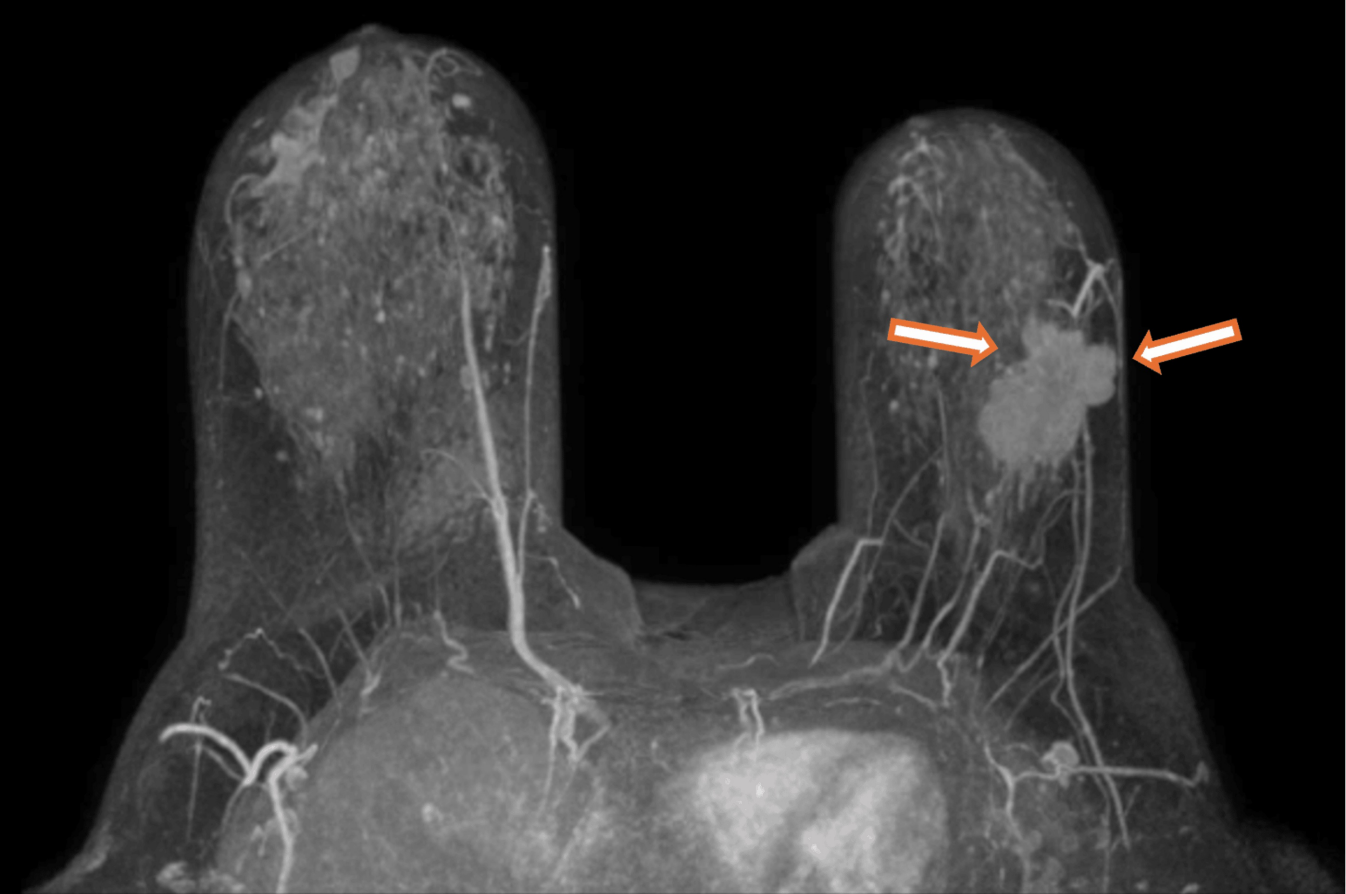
Breast MRI before neoadjuvant chemotherapy The two arrows indicate the primary tumor in the outer quadrants of the left breast MRI: magnetic resonance imaging
